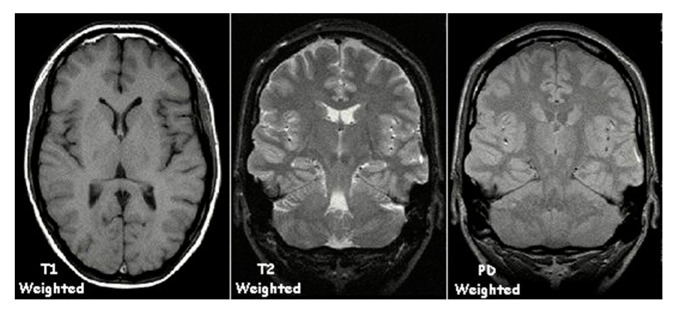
T1-weighted, T2-weighted, and proton density-weighted MRI images. Reproduced from https://en.wikipedia.org/wiki/Magnetic_resonance_imaging#/media/File:T1t2PD.jpg, accessed on 24 April 2022.
